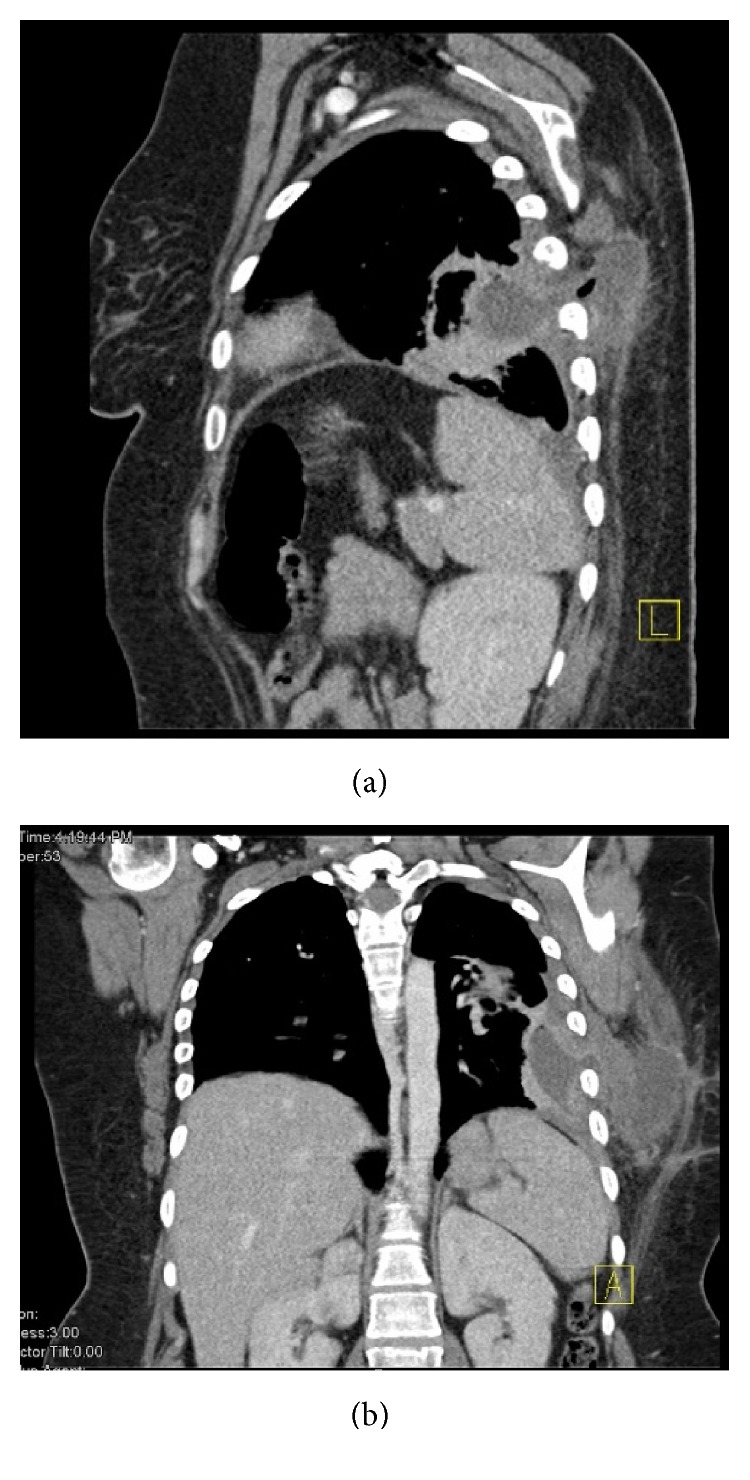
(a) Sagittal reconstruction of CT scan demonstrating the extension of the intrapleural collection into the subcutaneous tissue. (b) Coronal reconstruction demonstrating the same findings consistent with empyema necessitans.
